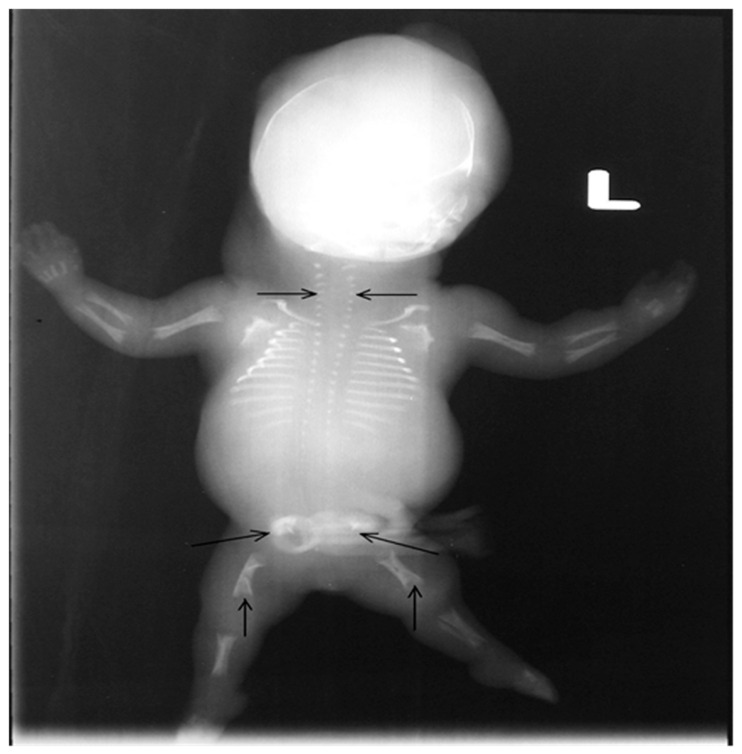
The post-mortem radiograph showed a normal ossified skull, short ribs, only ossified vertebral pedicles of the spine (horizontal arrow), short, long bones, hypoplastic ilium with a medial spike, and hypoplastic ischium (oblique arrow). The tubular bones were short and broad with flared and cupped metaphyseal margins (vertical arrow).
